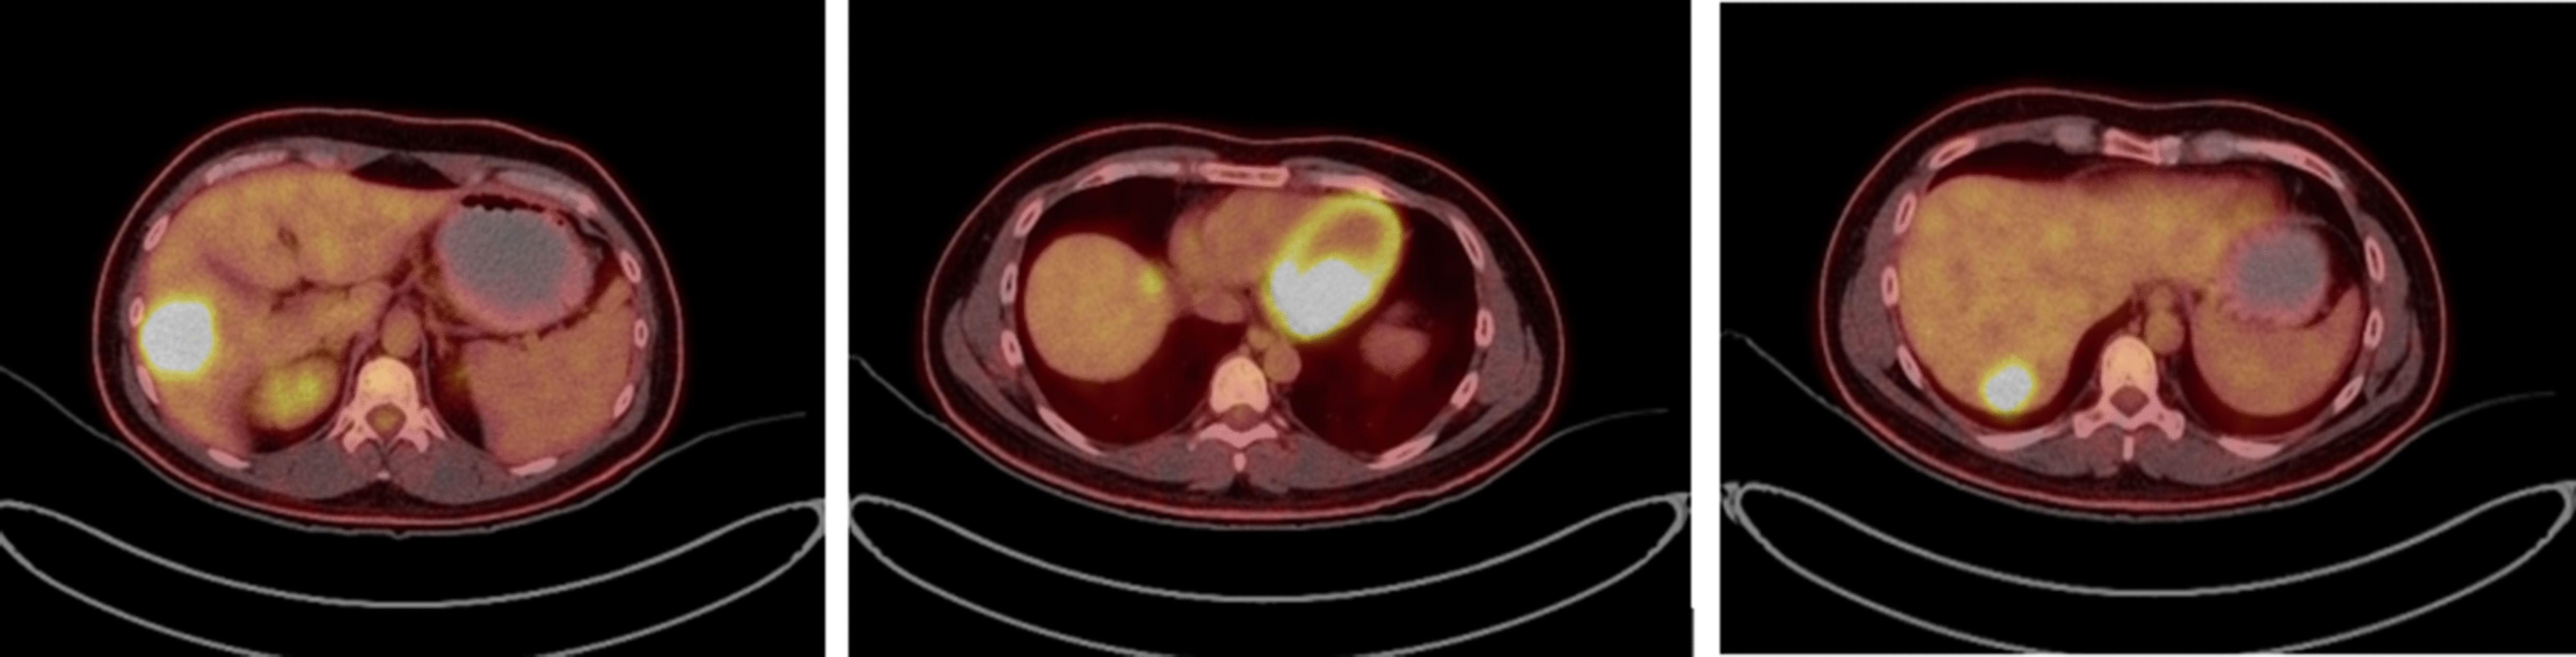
PET/CT scan showing the metastatic liver lesions
